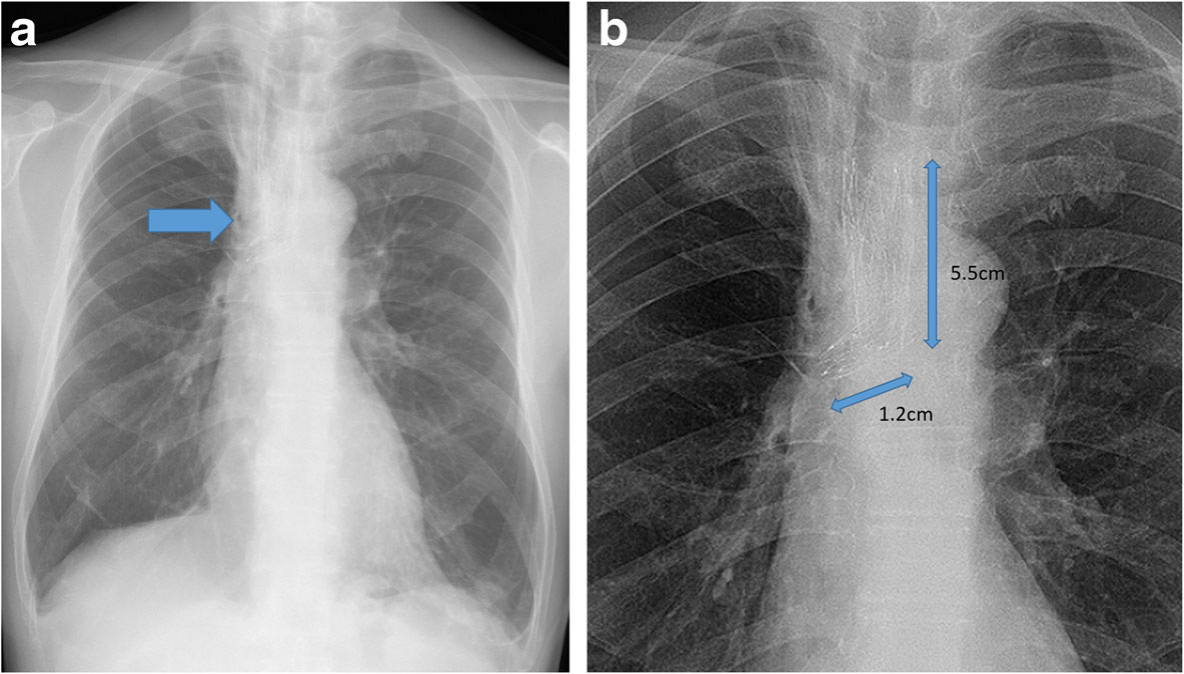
a An original chest X-ray is shown. b An enlarged X-ray with a high contrast shows the tracheal stent in place between the main bronchus and the main right bronchus
